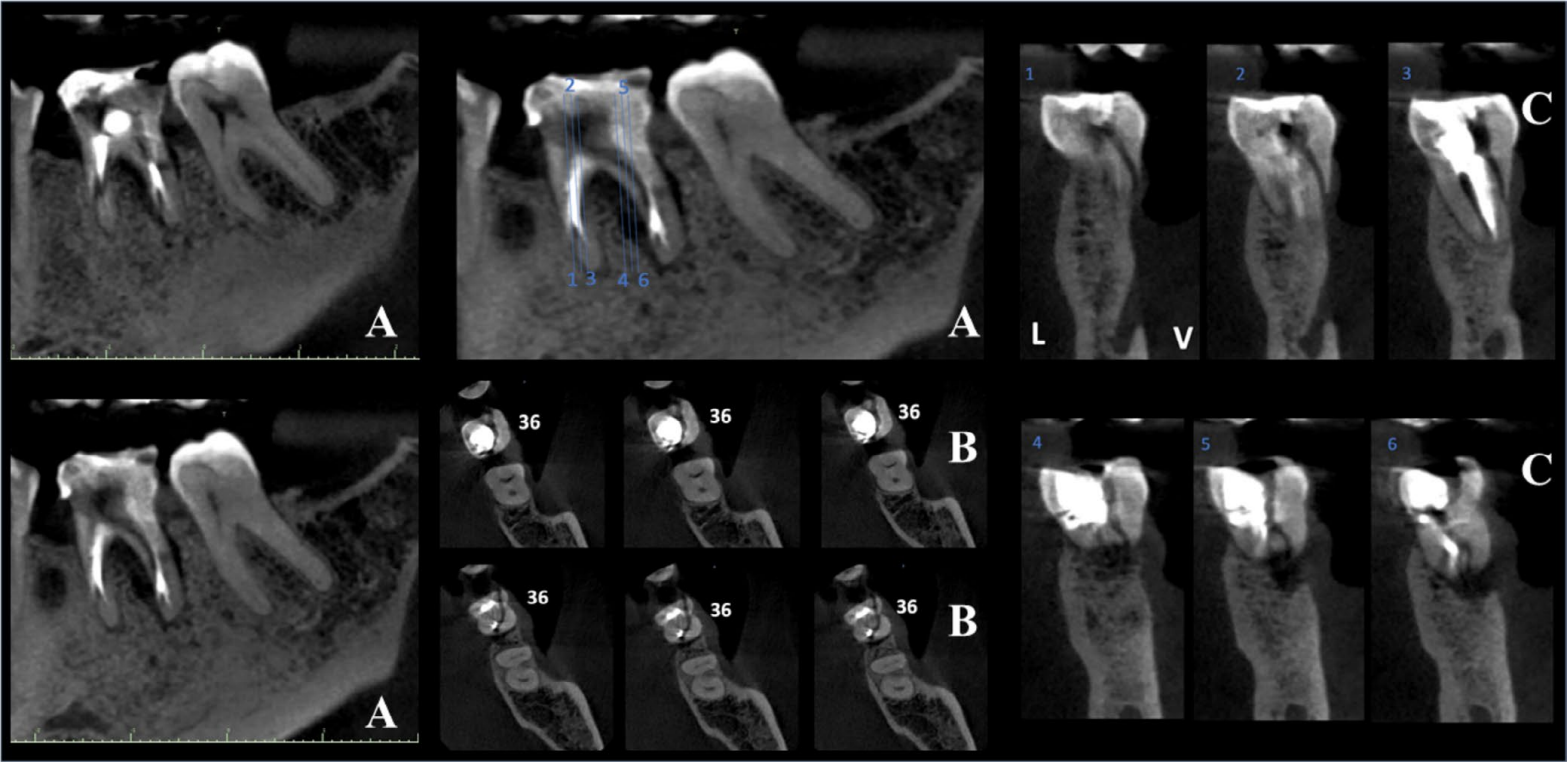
Cone beam computed tomography (CBCT) scan of the same case shown in Figure 2—interpretation stage 2. In addition to the periradicular radiolucency seen in the periapical radiograph, a vertical root fracture is visible in the left mandibular first molar, highlighting the superior detail provided by CBCT imaging in comparison to conventional radiography. Views: Panoramic reconstruction (A), axial slice (B), and oblique slice (C).
